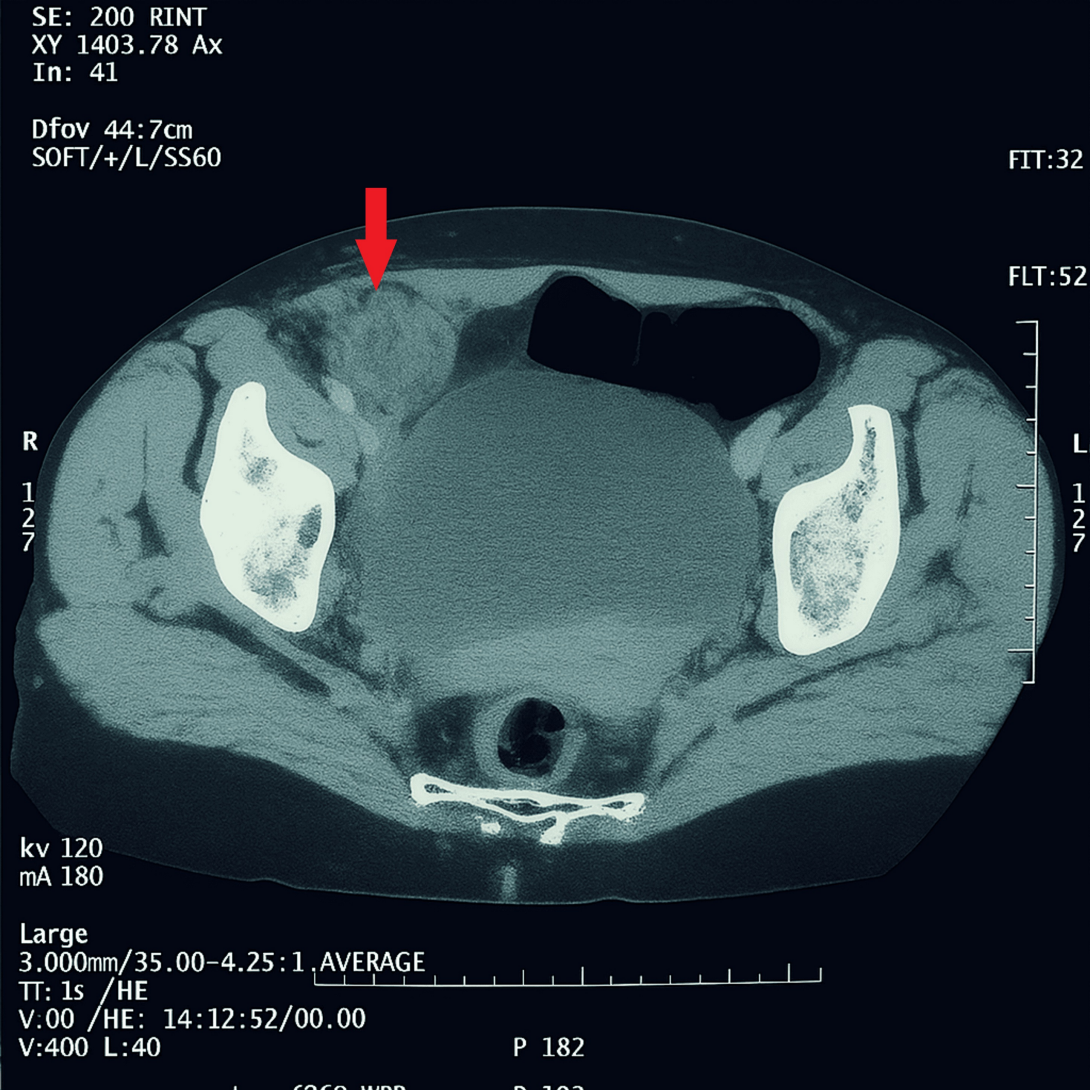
CT scan of the pelvic region showing a right laterocystic mass with a wall slightly enhanced by the contrast product (red arrow).
